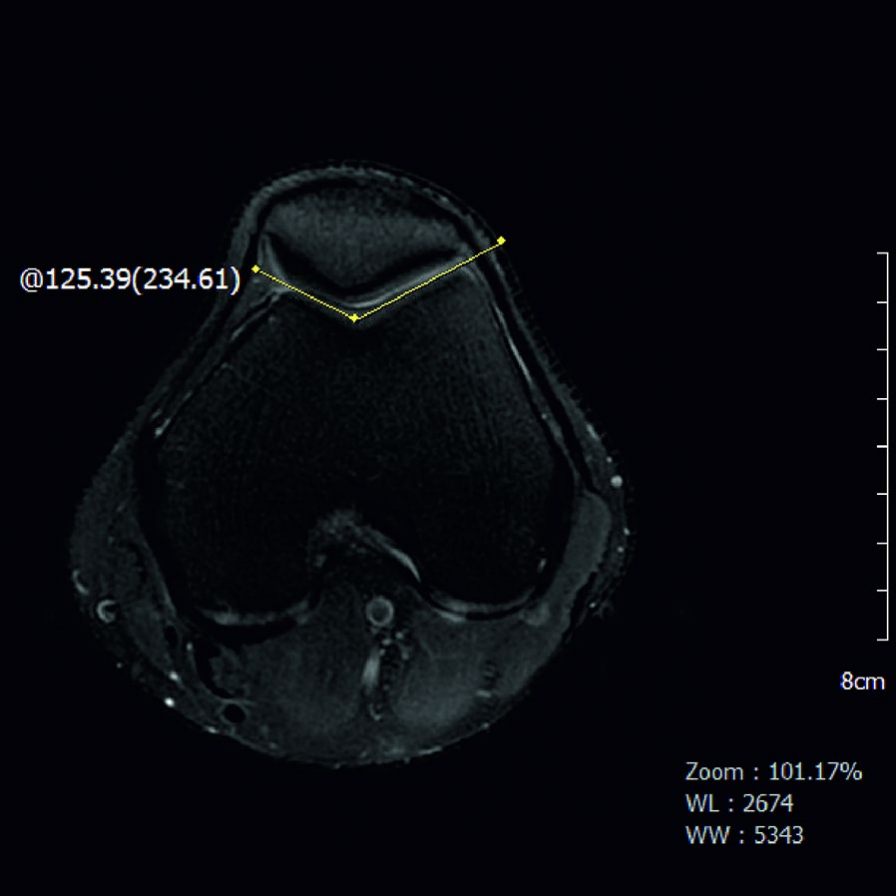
Axial proton density fat-saturated magnetic resonance imaging (PD-fatsat MRI) (a Sect. 3 cm above the knee joint). Note the normal trochlear groove and sulcus angle
